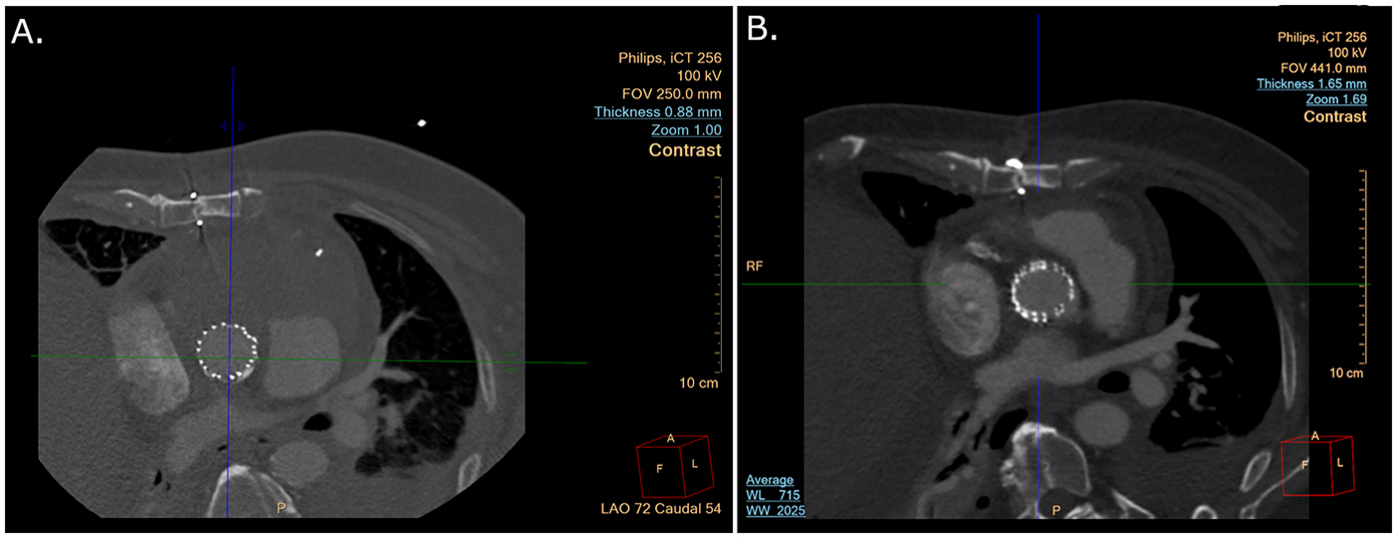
(A) CTA scan after endovascular pseudoaneurysm repair showing a large anterior mass. (B) CTA scan after endovascular pseudoaneurysm repair and hemisternotomy to remove blood clot and patch the aorta. CTA, computed tomography angiography.
